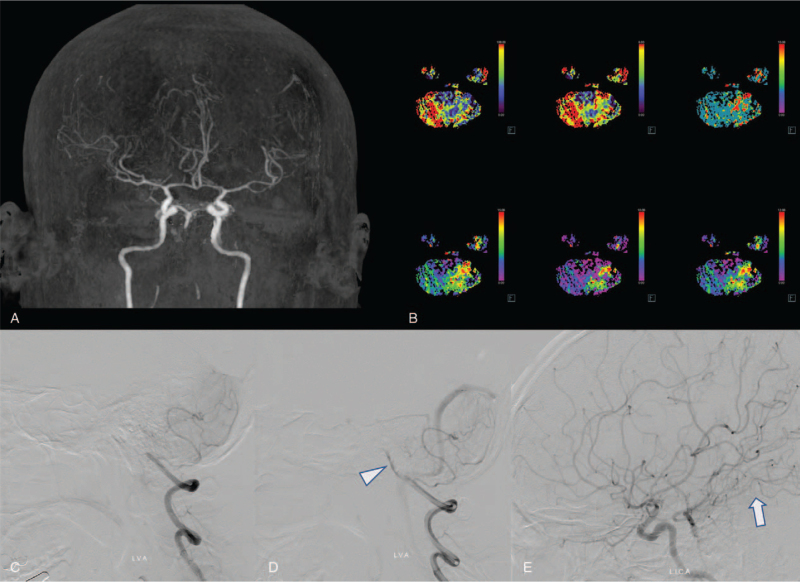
Brain CT angiography showing complete occlusion of the proximal portion of the vertebrobasilar artery (A). Perfusion CT showing a perfusion anomaly at the left cerebellum (B). Initial transfemoral cerebral angiography showing complete occlusion of the vertebrobasilar artery with thrombus filling (C). Spontaneous recanalization of the occluded vessel without thrombectomy (D, arrowhead). Follow-up angiography showing good blood supply in the posterior circulation, indicating a good collateral flow of the distal portion of the basilar artery (E, arrow). CT = computed tomography.
